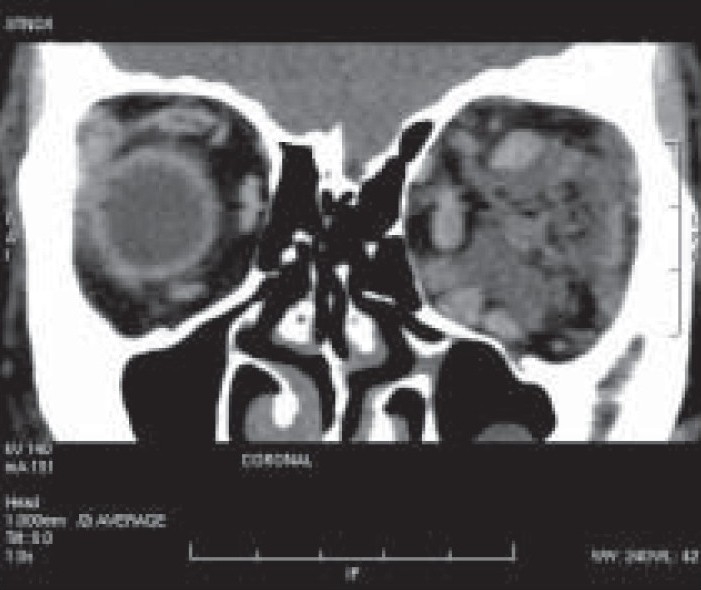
Reformatted coronal post-contrast thin section CT scan shows the retrobulbar unencapsulated non-enhancing intraconal lesions
